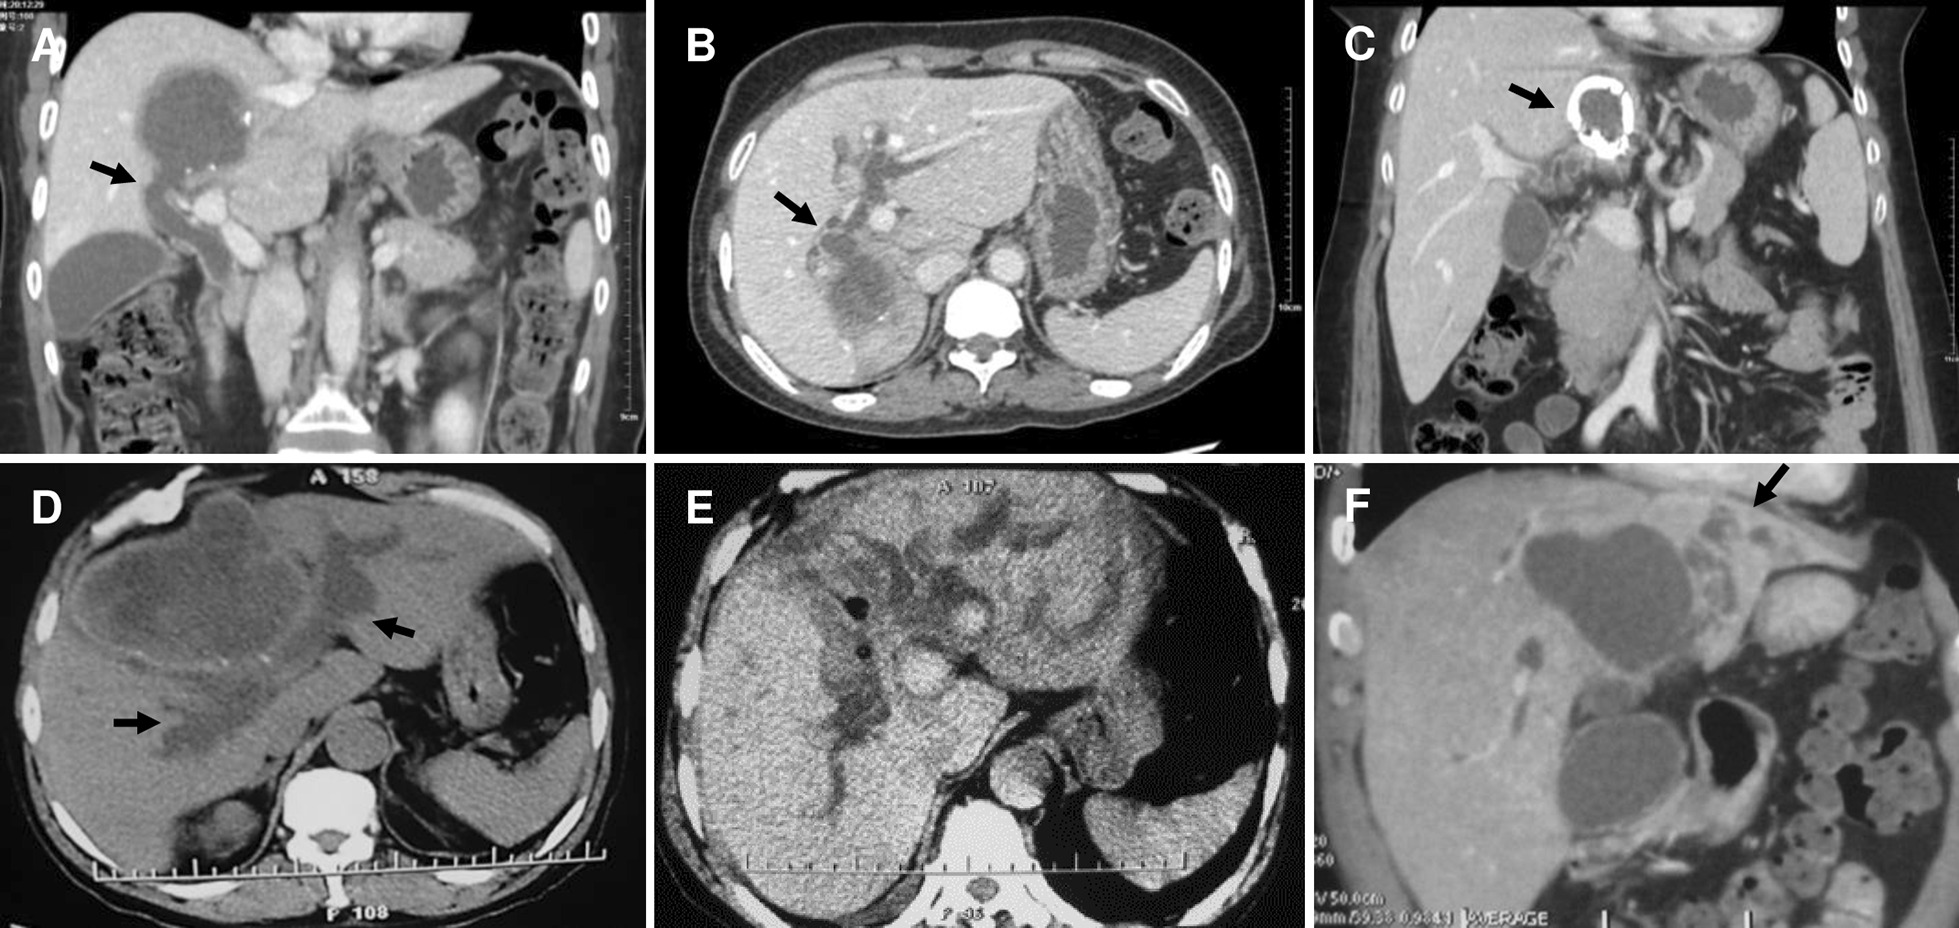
CT presentations of frank cysto-biliary communications. a Cysto-biliary communication (arrow) at right supra section and debris-filled common bile duct; b Cysto-biliary communication (arrow) at right posterior lobe; c Capsule calcified cyst (arrow) interlinking with left hepatic duct; d Medial lobular cyst presenting cysto-biliary communication to major hepatic ducts form both sides (arrows); e Severe left lateral lobe liver damage caused by cysto-biliary communication; f Hepato-atrophy (arrow) of left lateral lobe led by cysto-biliary communication
